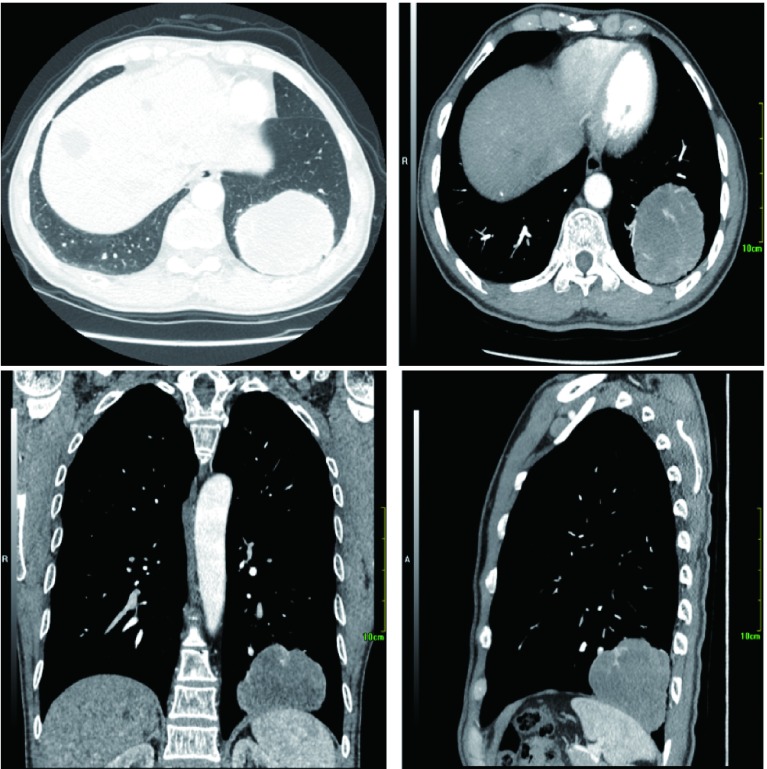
胸部CT显示左肺下叶基底段可见巨大类圆形肿块影，大小约7 cm×8 cm，密度欠均匀，增强扫描双期CT值分别为38 HU-58 HU、31 HU-55 HU，其内可见血管穿行，边缘呈浅分叶，境界清晰，相应支气管截断，局部胸膜肥厚。 Chest CT shows a heterogeneous well-defined peripheral mass in the basal segment of the left lower lobe. Size of the tumor is approximately 7 cm×8 cm and the dual-phase CT values of enhanced scan are 38 HU-58 HU and 31 HU-55 HU, respectively. The tumor is shallowly lobulated with blood vessels passing through, bronchial truncation and local pleural hypertrophy. CT: computed tomography.
